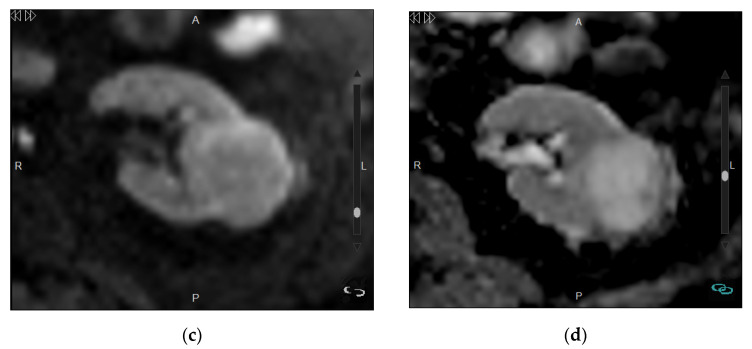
Oncocytoma in a 57-year-old man. (a) Axial T2-weighted MRI shows a nodular mass in the left kidney with heterogeneous but mainly hyperintense signal; (b) Axial contrast-enhanced T1-weighted image shows heterogeneous enhancement with intratumor hypoenhancing areas; (c) Axial diffusion-weighted image with high b = 1000 (d) and Apparent Diffusion Coefficient (ADC) map show absence of restriction.
